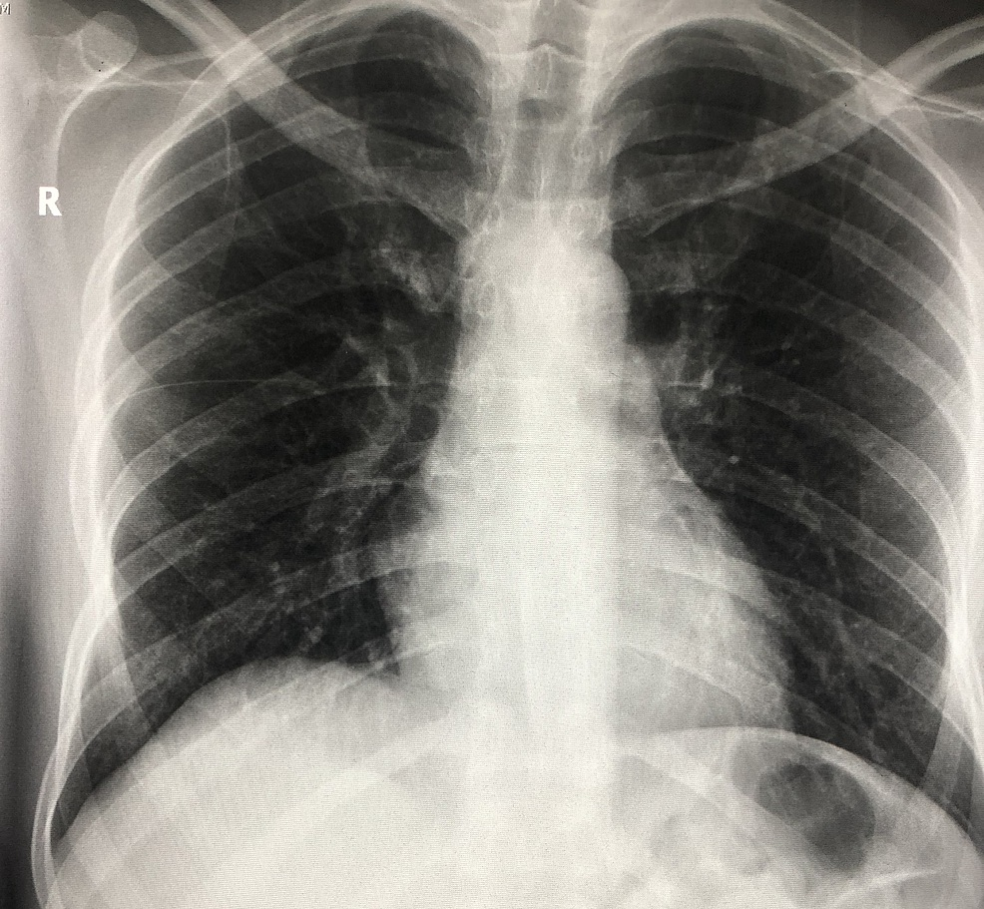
Normal chest X-ray - no signs of bronchial Infiltrates
